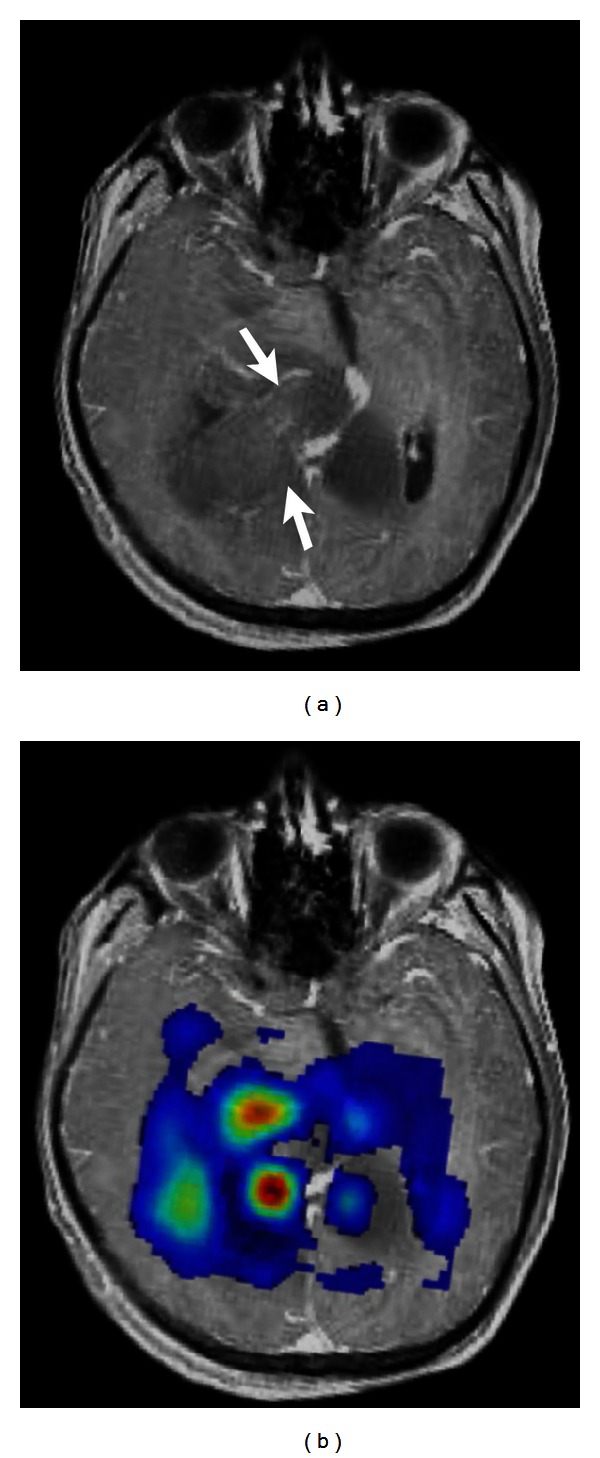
(a) Contrast-enhanced T1-weighted image depicts a glioblastoma involving the genu of corpus callosum. The arrows point two hot spots (targets) for stereotactic biopsy based on the regions of increased Cho/Cr ratios ((b) Cho/Cr map).
